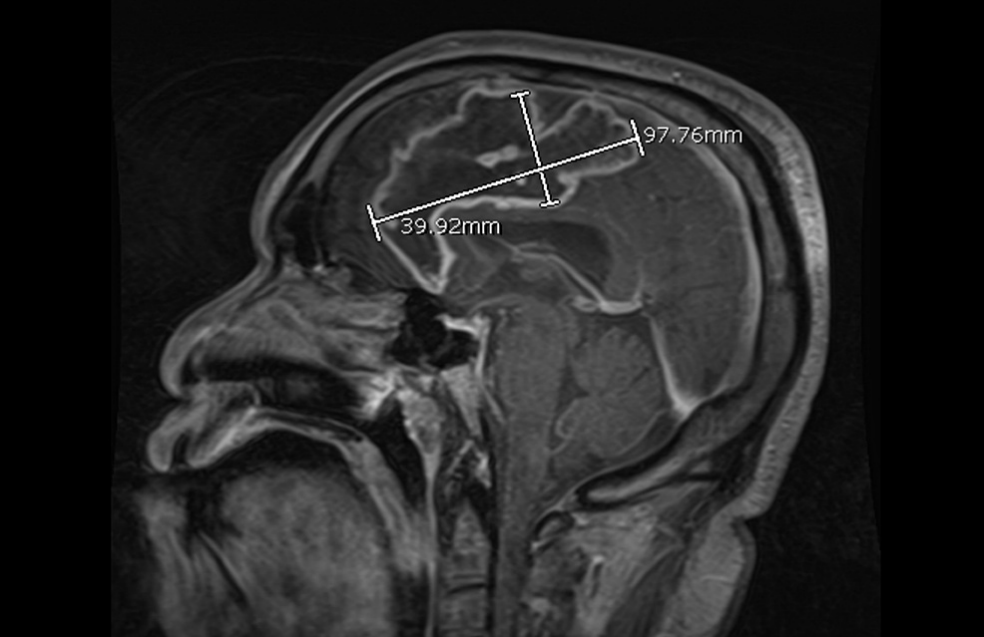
Contrast-enhanced MRI showing a 9.7 x 3.9 x 2.6 cm (98 cc) ring enhancing lesion of the left frontal lobe in the prior area of infarction. MRI, magnetic resonance imaging
